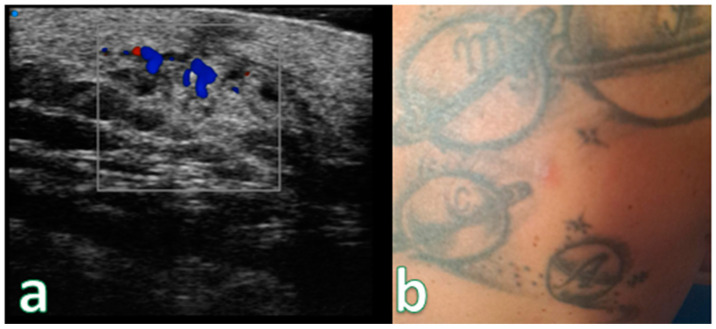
(a) Examination performed with a very high-frequency ultrasound probe at 48 MHz, showing iso-hypoechoic lesions with nodular and multilobulated morphology and irregular margins infiltrating the epidermal and subepidermal tissue at a single point (focal). (b) Reddish, indistinct, slightly exofitic nodule in the lumbar region. Color Doppler analysis showing no significative increase in the signals. Pathological assessment showed B-Cell Lymphoma (recurrence).
